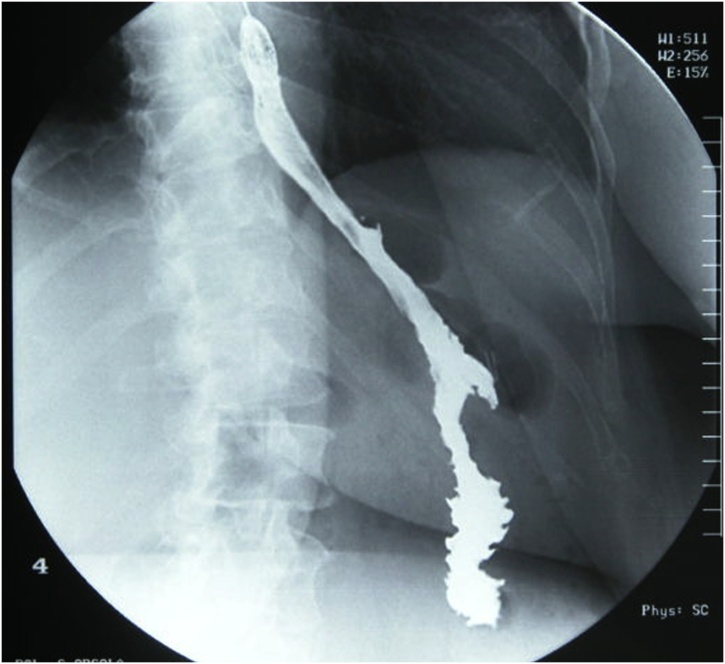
X-ray with Gastrografin demonstrating the normal progression of dye without pathological findings.
